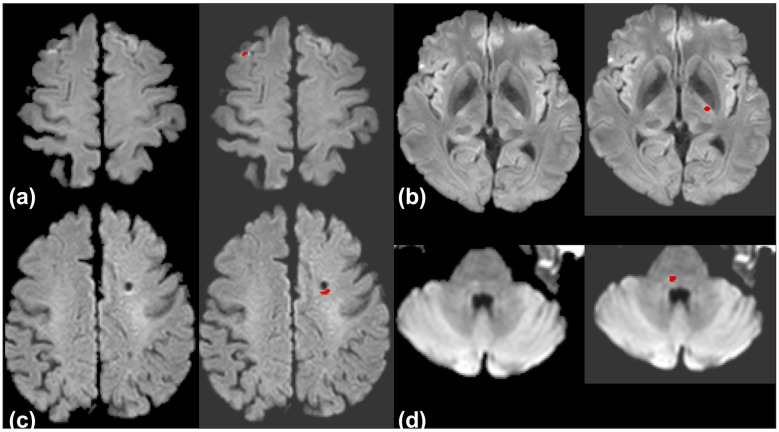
Representative cases of common false-positive lesions detected by the AI model. (a) Right frontal cortex: susceptibility artifact at the brain-skull interface. (b) Posterior limb of the left internal capsule: accentuated T2-weighted signal of normal anatomy. (c) Left frontal white matter: susceptibility artifact from adjacent microbleeds. (d) Right pons (medial lemniscus): hyperintensity related to small vessel disease.
